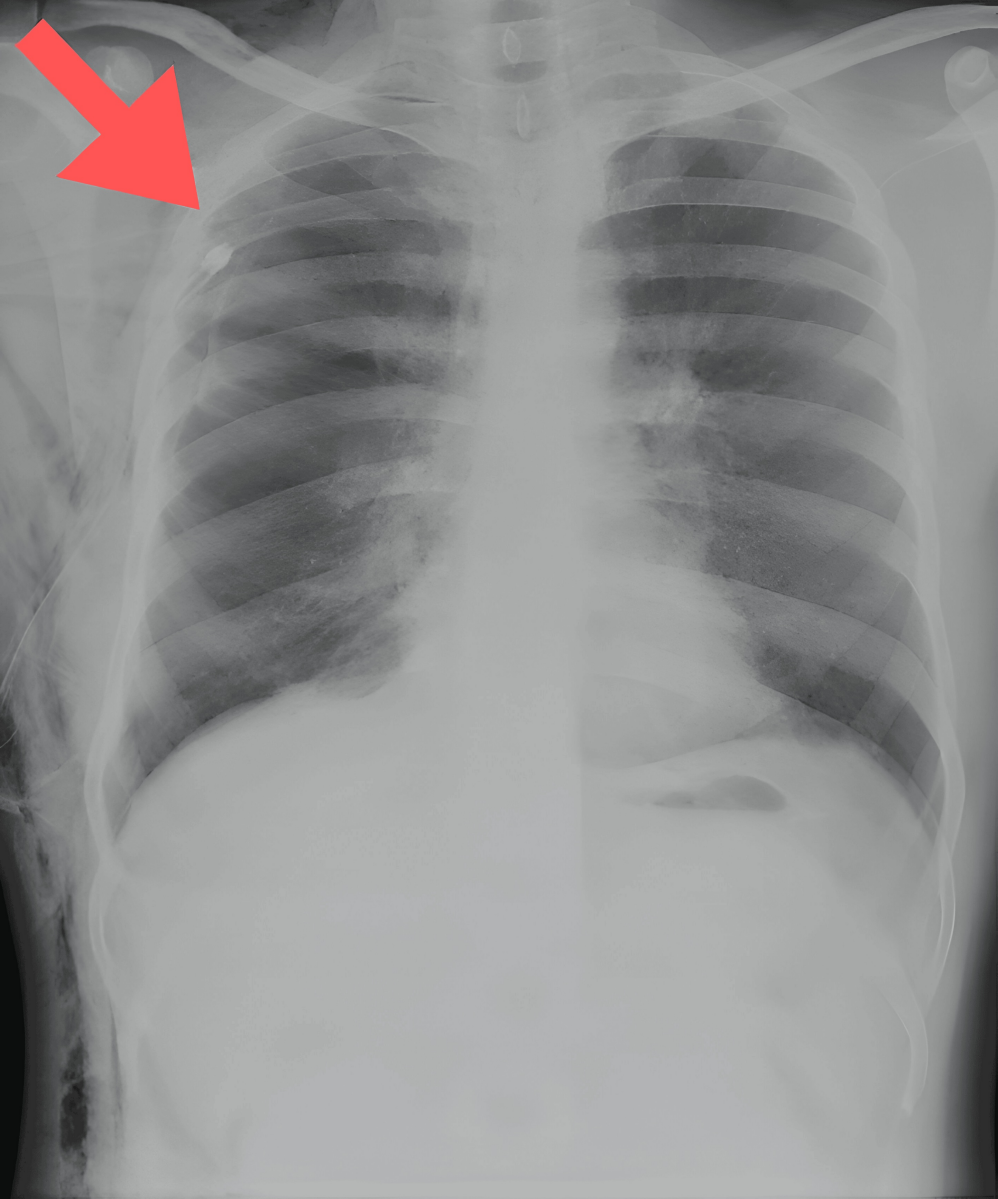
Chest X-ray post-intercostal drainage tube insertion (arrow indicating the intercostal drainage tube).
